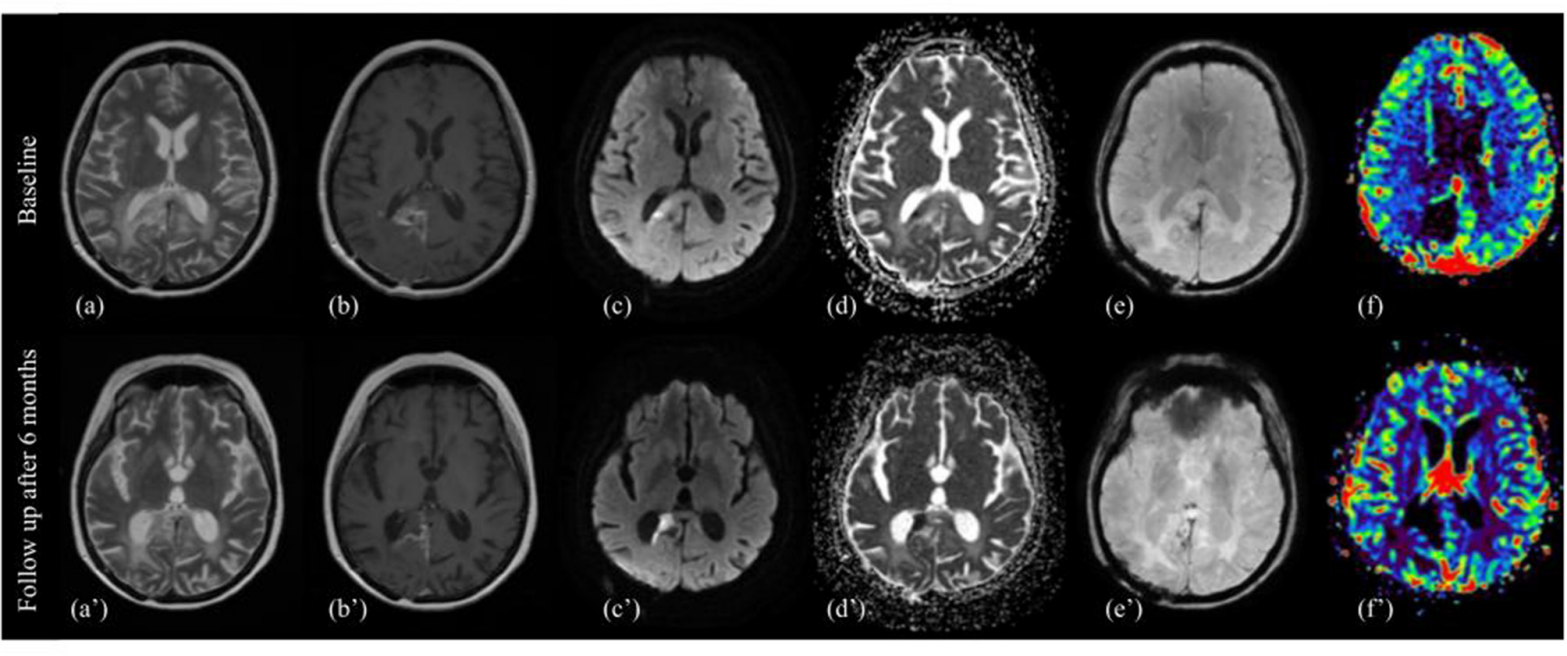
Pattern B. MRI changes in recurrent high grade glioma under regorafenib, pattern B. MRI scans performed at baseline (top image) and after six months (bottom image) from the first administration of REG therapy. T2w images (a,a'), contrast enhanced T1w images (b,b'), diffusion-weighted b1000 (c,c'), apparent diffusion coefficients (ADC) map (d,d'), SWI images (e,e'), and color-coded perfusion map (f,f') are shown from left to right. On 6-month follow-up MRI, the previously enhanced tumor component showed a dramatically absence of CE, diffusion restriction on DWI and ADC map, and decrease signal on corresponding T2 image, surrounding by a thin hypointense rim on SWI, and peripheral contrast enhancement on T1-wi after gadolinium. There was also a decrease of peritumoral edema.
